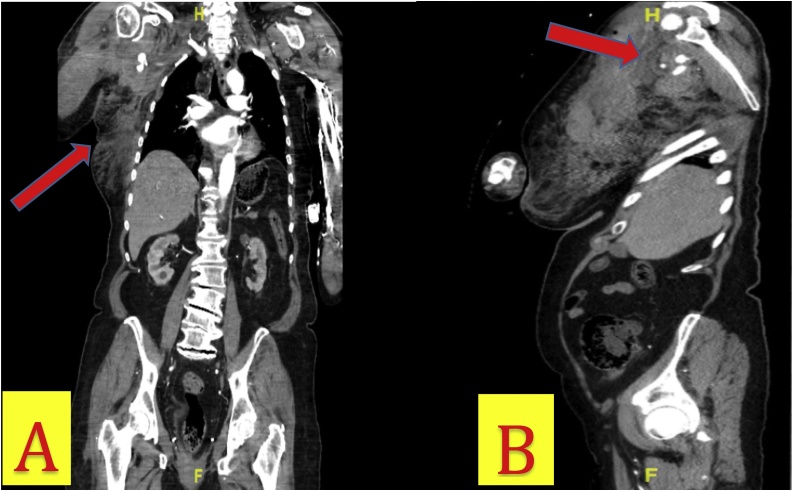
A) CT Angiography of the chest and abdomen (coronal view) demonstrating right subclavian artery dissection, 2.4-cm axillary artery bilobed pseudoaneurysm, possible proximal brachial artery injury, and large right chest wall hematoma. B) CT Angiography of the chest and abdomen (sagittal view) showing large right shoulder hematoma and pseudoaneurysm in proximity to the humeral head of the right post-reduction. Pseudoaneurysm is positioned in the inferior location to the gleno-humoral joint.
